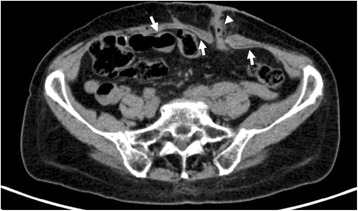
Axial abdominal CT image without intravenous contrast. It can be appreciated the location of the mesh (*arrows*) fitted around the bowel (*arrowhead*)
